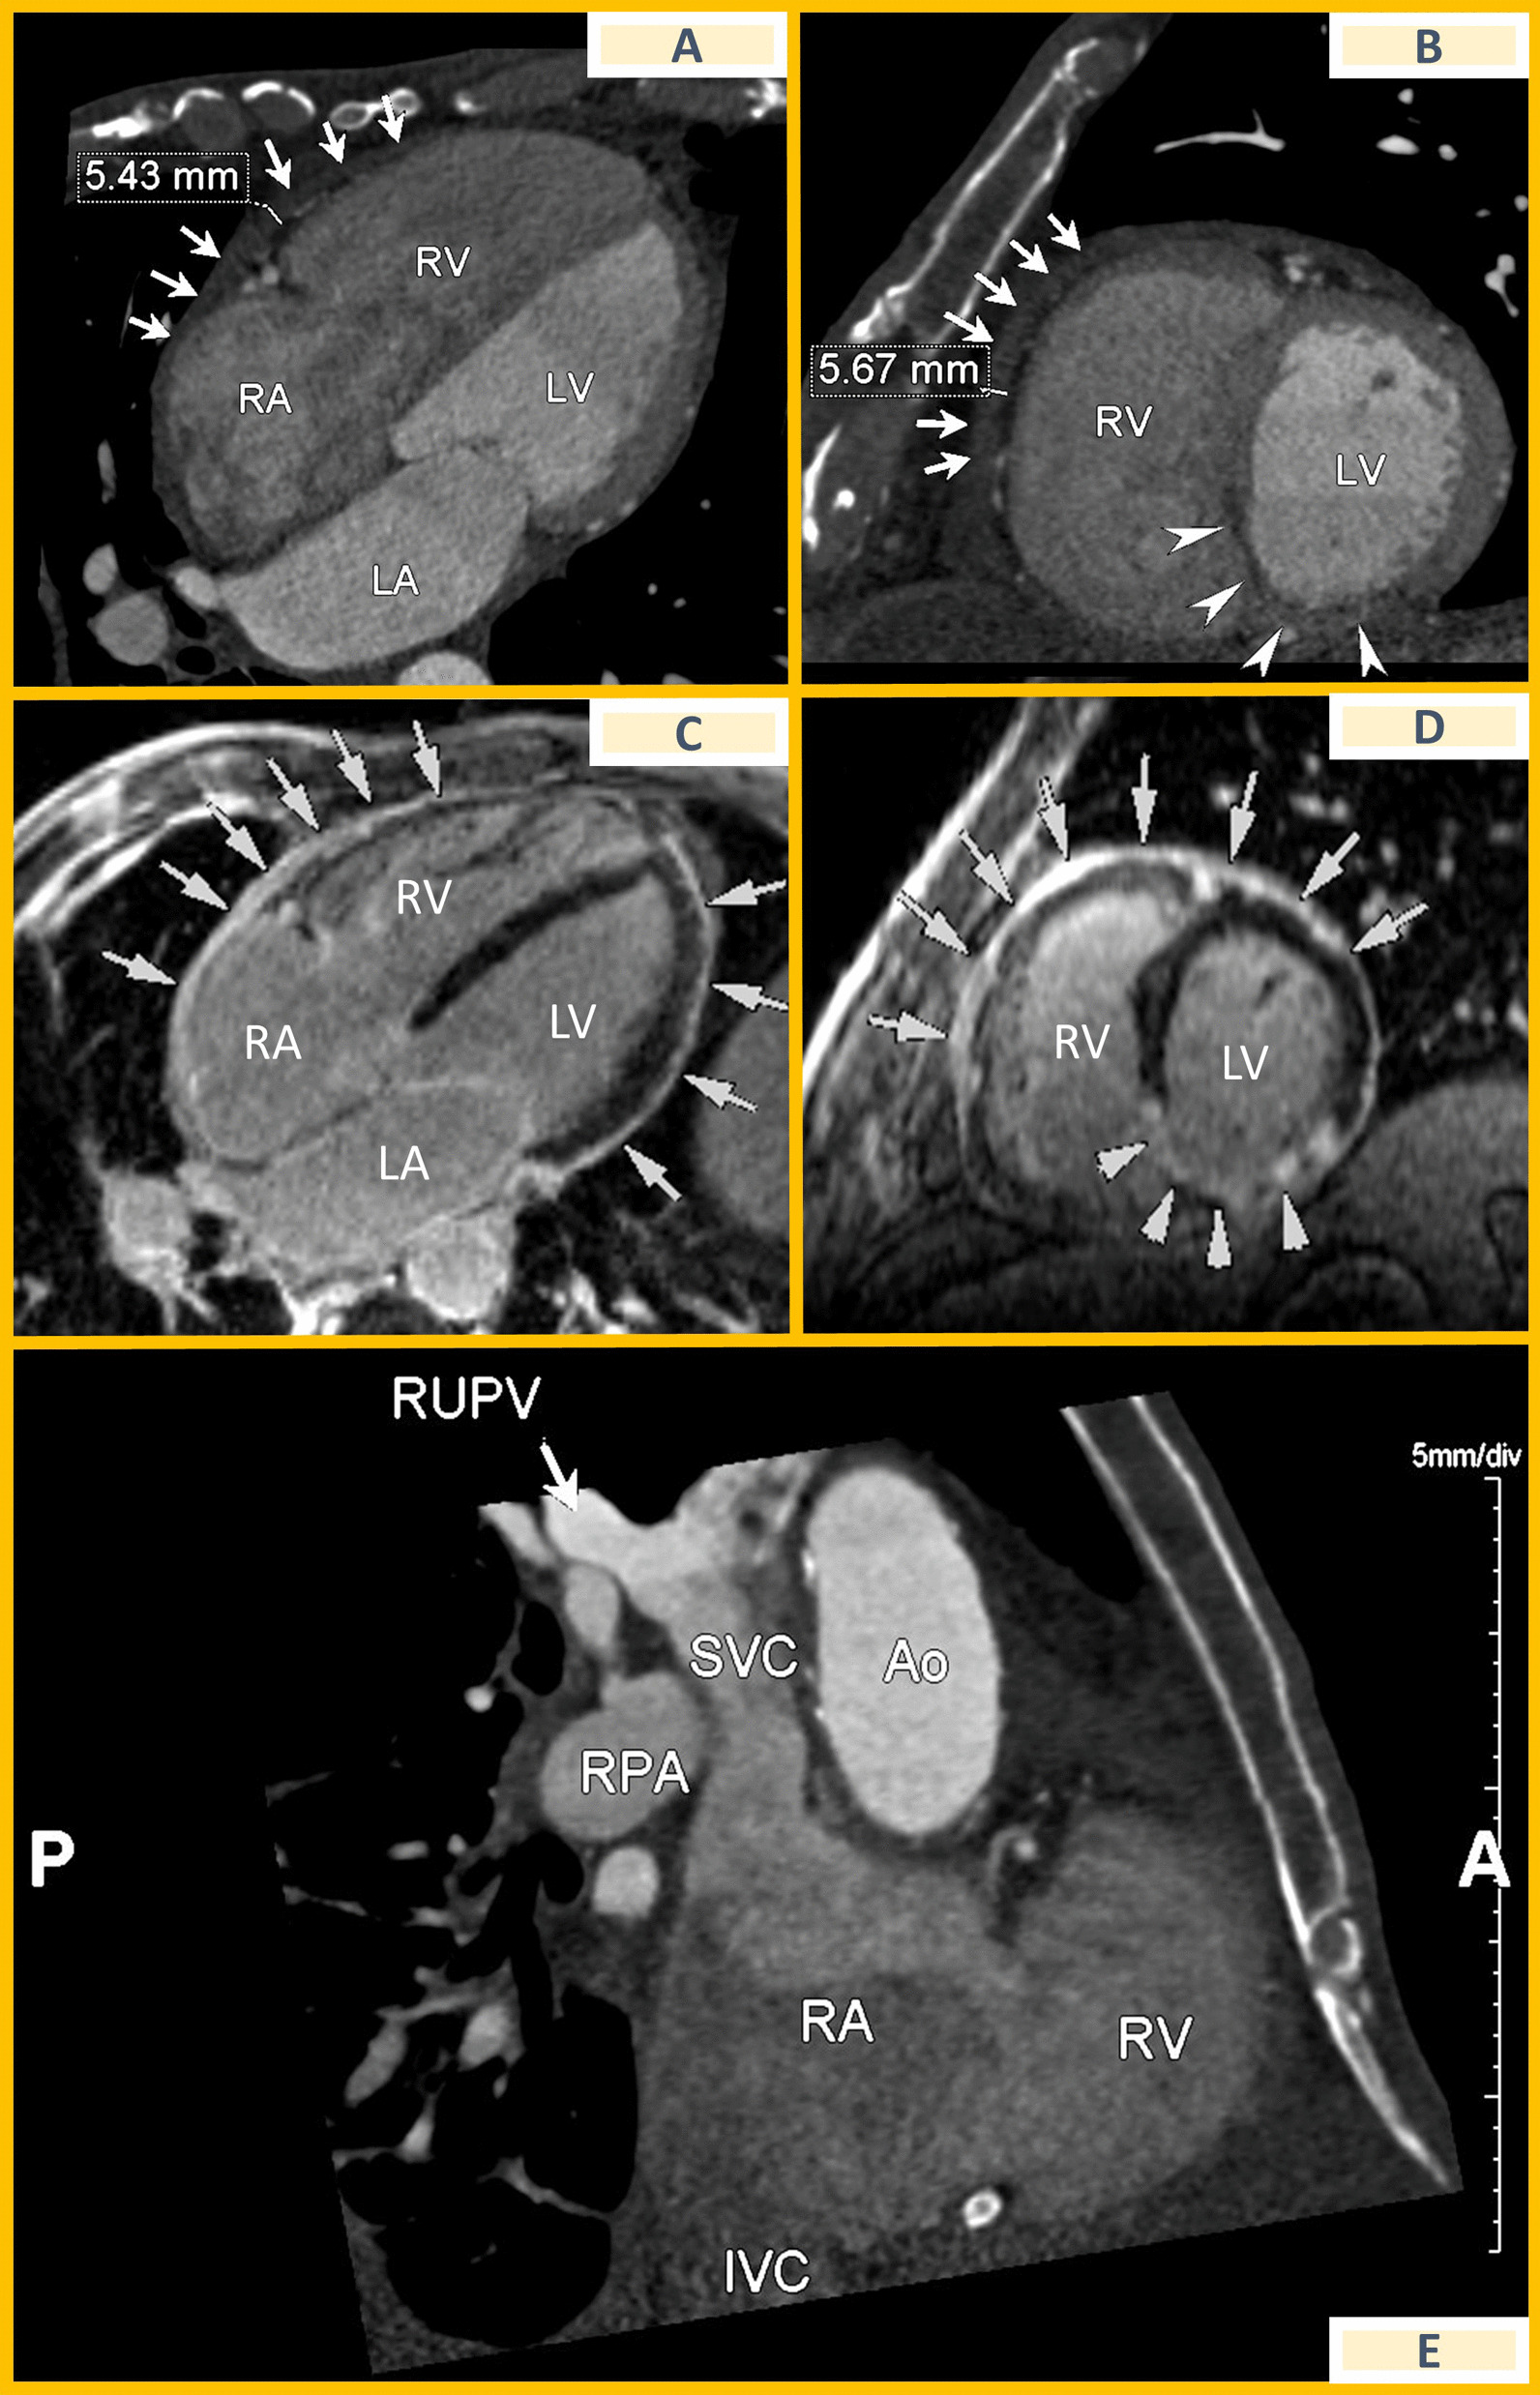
A Computed tomography showing thickened pericardium (arrows); B computed tomography revealing pericardial thickening (arrows) and inferior myocardial-wall scar (arrowheads); C magnetic resonance revealing pericardium late-gadolinium enhancement (arrows); D magnetic resonance revealing pericardial thickening (arrows) and inferior myocardial-wall scar (arrowheads); E computed tomography showing abnormal pulmonary venous return. Ao = aorta; IVC = inferior vena cava; LA = left atrium; LV = left ventricle; RA = right atrium; RPA = right pulmonary artery; RUPV = right upper pulmonary vein; RV = right ventricle; SVC = superior vena cava
